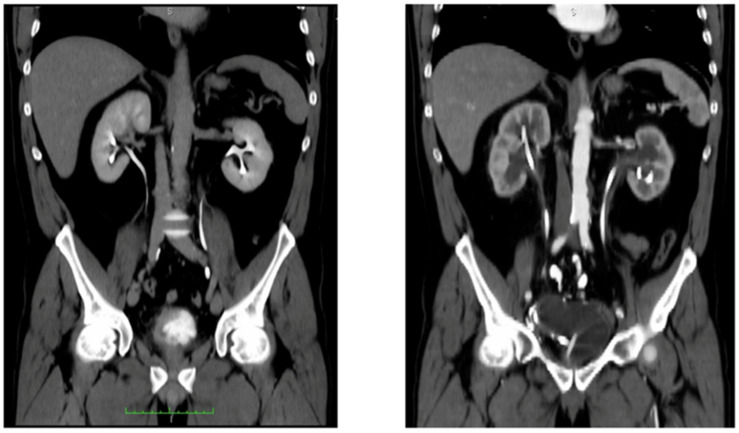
(Left) CT-urography showing pathological bladder thickening, later diagnosed as MIBC, with regular upper urinary tracts. (Right) CT scan, arterial phase, taken after development of bilateral ureteral-ileal stenosis with hydronephrosis treated at the previous institution with ureteral stentin. Green scale bar: cms.
